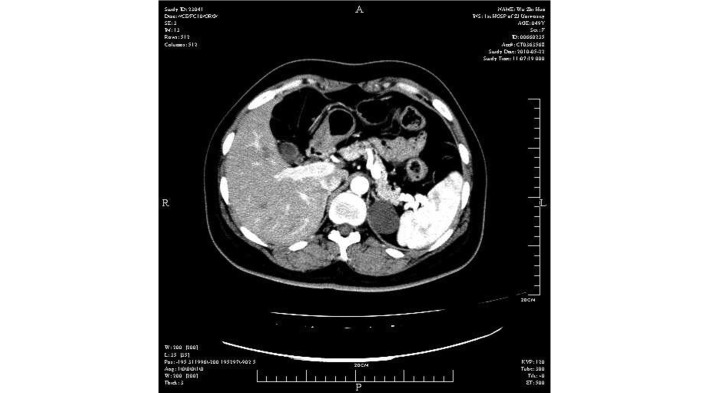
Contrast-enhanced computed tomography (CT) scan revealing a low density mass in the left adrenal gland.
